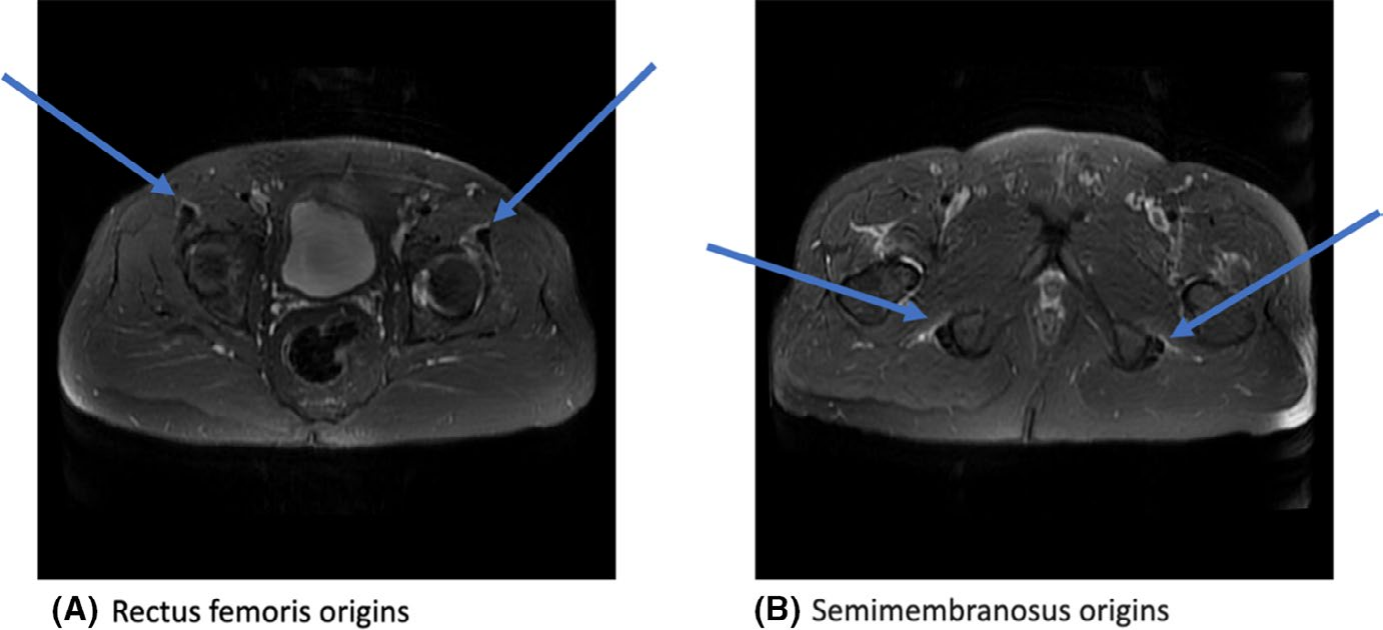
Initial MRI pelvis with and without contrast with nonspecific edema and enhancement along the bilateral semimembranosus and rectus femoris origins
